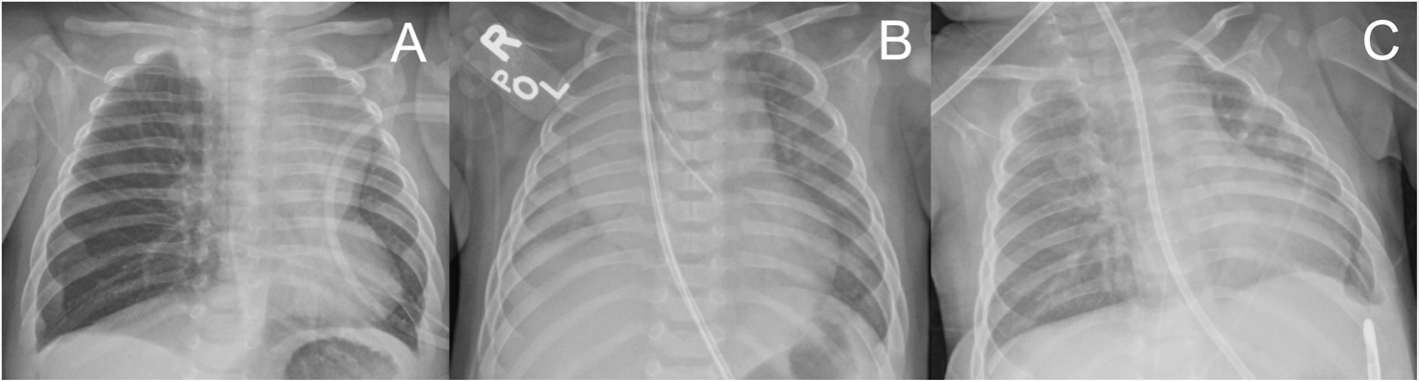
Serial chest x-rays from (A) initial presentation, (B) after left-mainstem intubation, and (C) after 10 days of treatment and extubation
